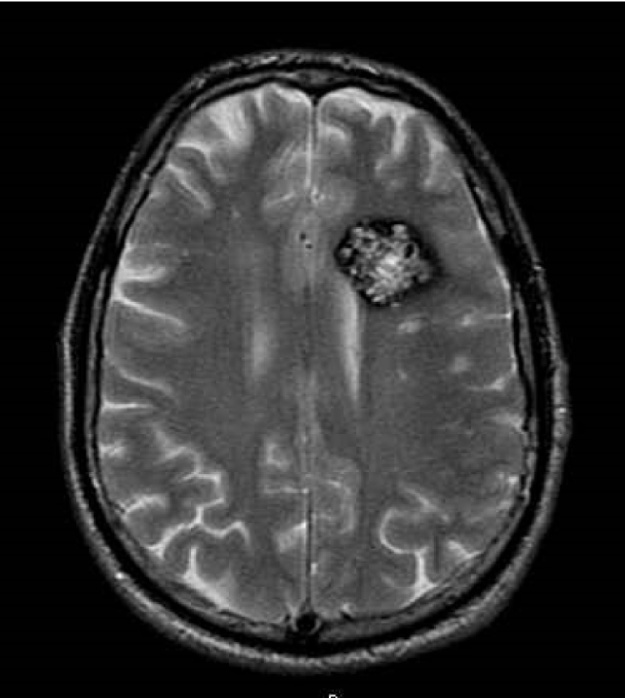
MRI appearance of a Cerebral Cavernous Malformation. Axial T2 FSE MRI of a CCM lesion in an affected patient (*image courtesy of Dr. Maria Consuelo Valentini, “Città della Salute e della Scienza” University Hospital of Torino, Italy*).
